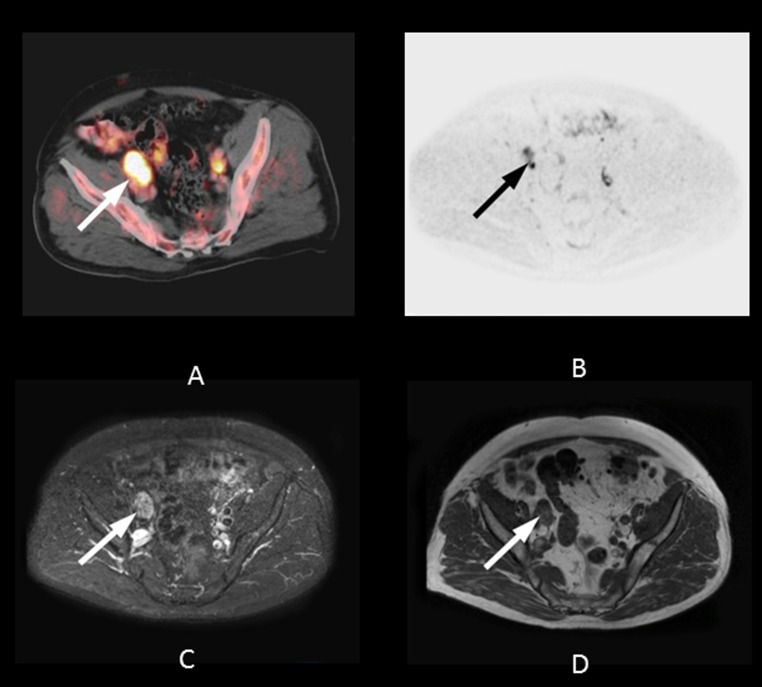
74-year-old patient with lymph node metastases six years after radical prostatectomy (initial Gleason Score: 7) The PSA level at the time of imaging was 21.0 ng/mL. A regression in lesion size after pelvic irradiation confirmed the malignant nature of the lesions. A. Axial PET/CT fusion image showing focal 11C-choline uptake in the area of the right external iliac vessels (white arrow, SUV max 3.6). The lesion shows focal signal elevation on both the 500 ms b-image using DWI B. (black arrow) and the STIR image C. (white arrow). D. Axial fusion T1w image showing enlarged lymph nodes (20 mm) close to the right external iliac vein.
